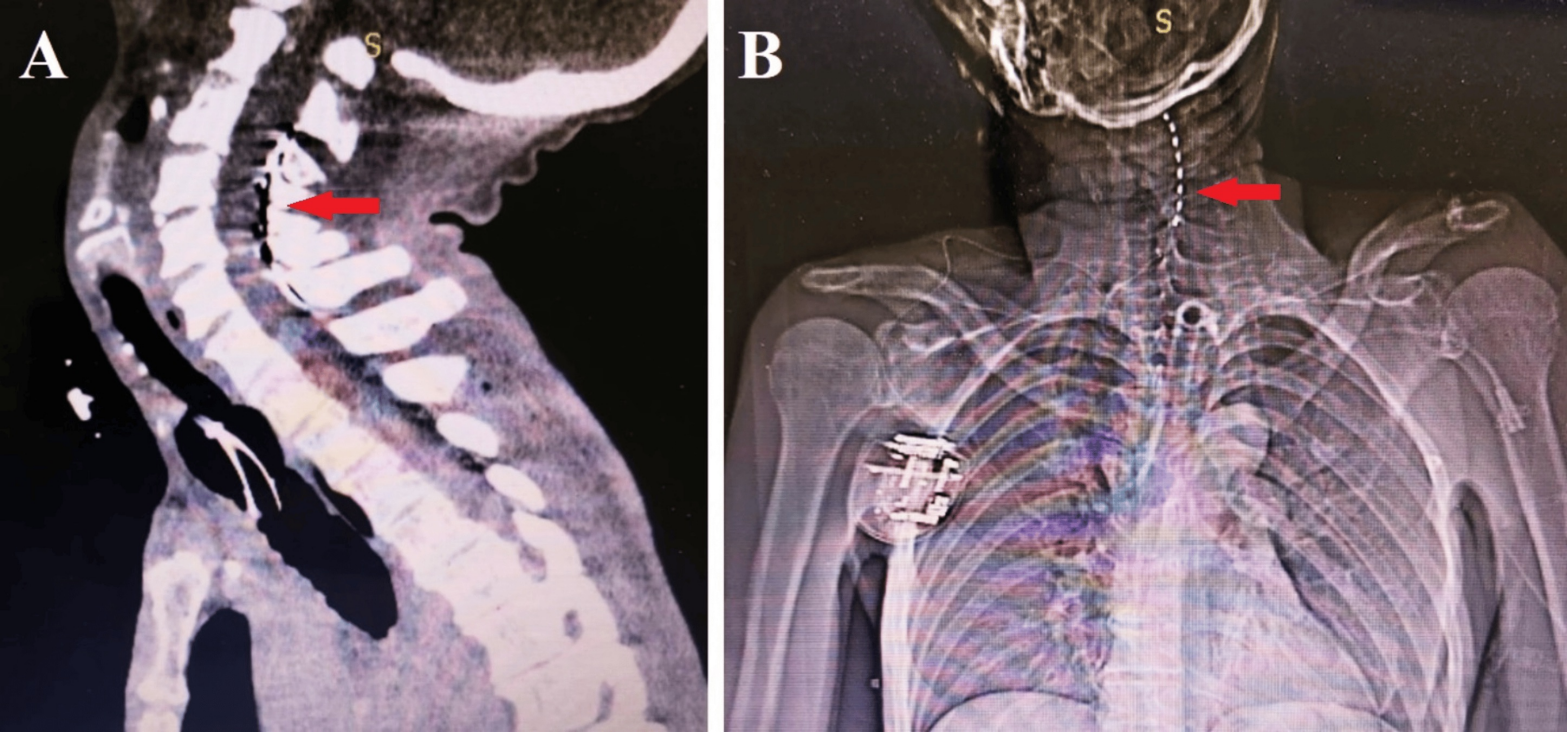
Postoperative CT (A) and X-ray (B) showing the epidural spinal cord electrode (red arrows) at the C3-C5 level.
